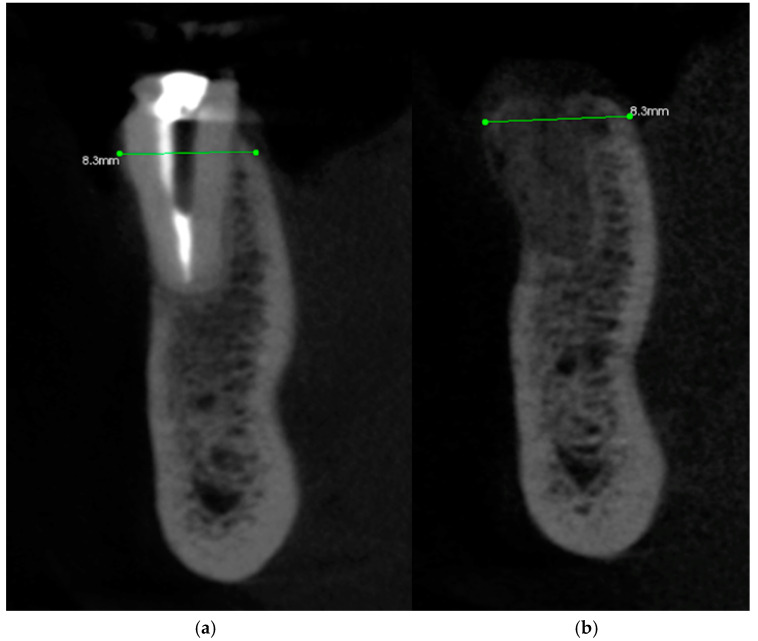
(a) CBCT images before the surgery (sagittal section) and (b) CBCT images after 4 months (sagittal section).
